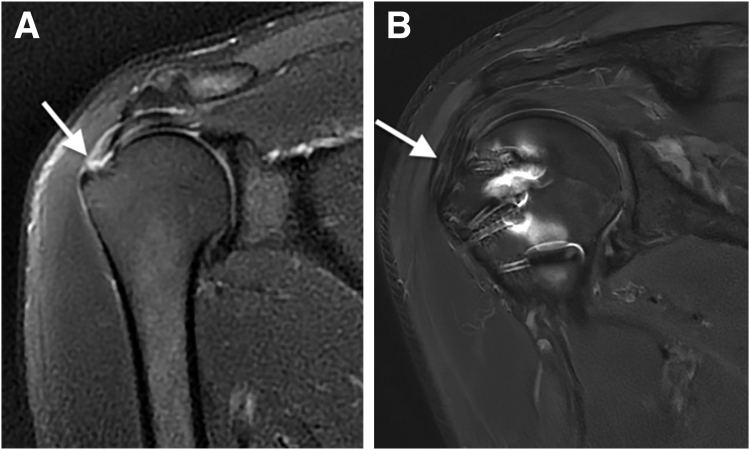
Magnetic resonance imaging (T2 fat-supression frontal view) of right shoulder showing full-thickness tear of supraspinatus tendon (arrow) (A) and healed supraspinatus tendon (arrow) (Sugaya stage 1) at 12 months after arthroscopic repair (B).
